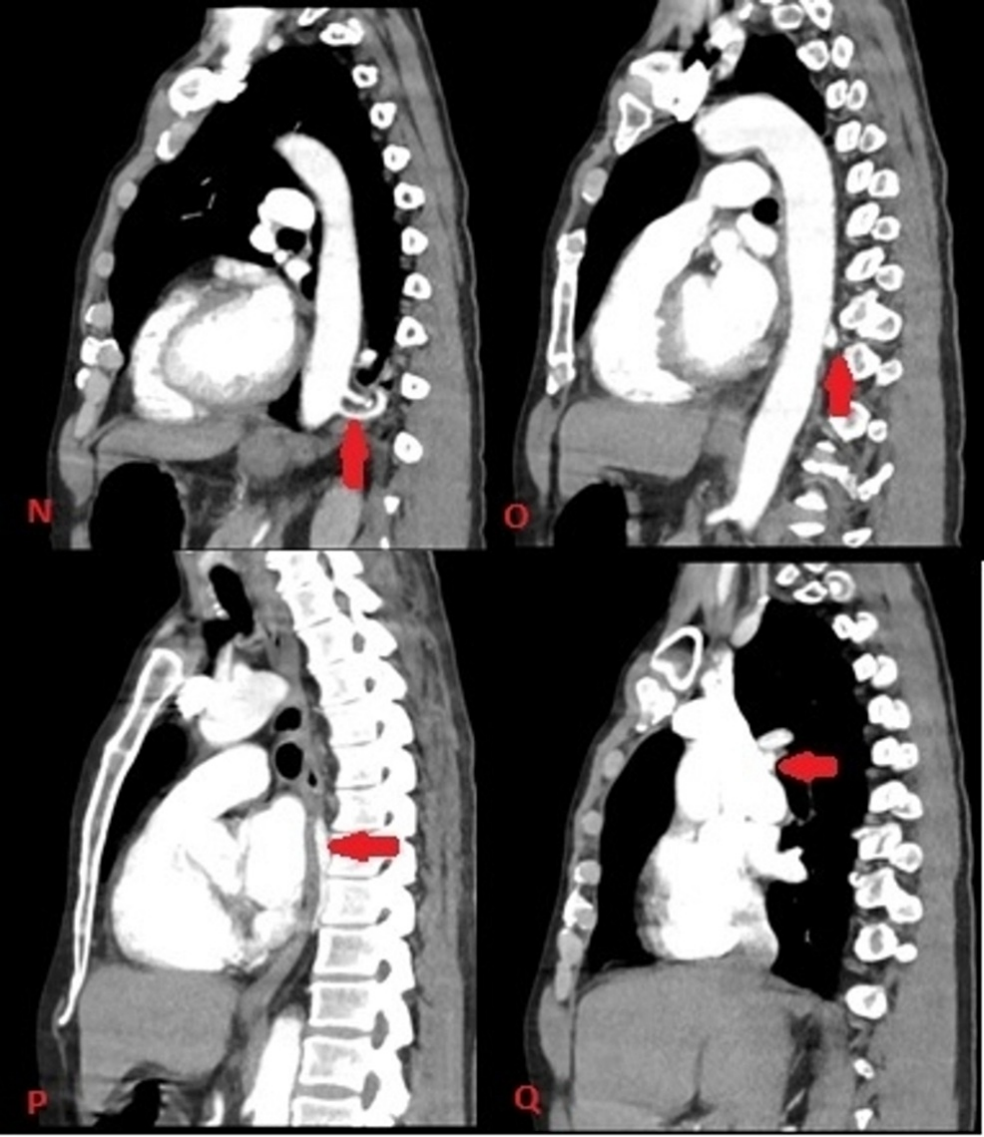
CT of the chest with contrast in the sagittal plane with red arrow showing feeding artery arising from the aorta (Image N), the pulmonary vein coursing around the side of aorta (Image O and P) and ultimately joining the azygos system (Image Q)
